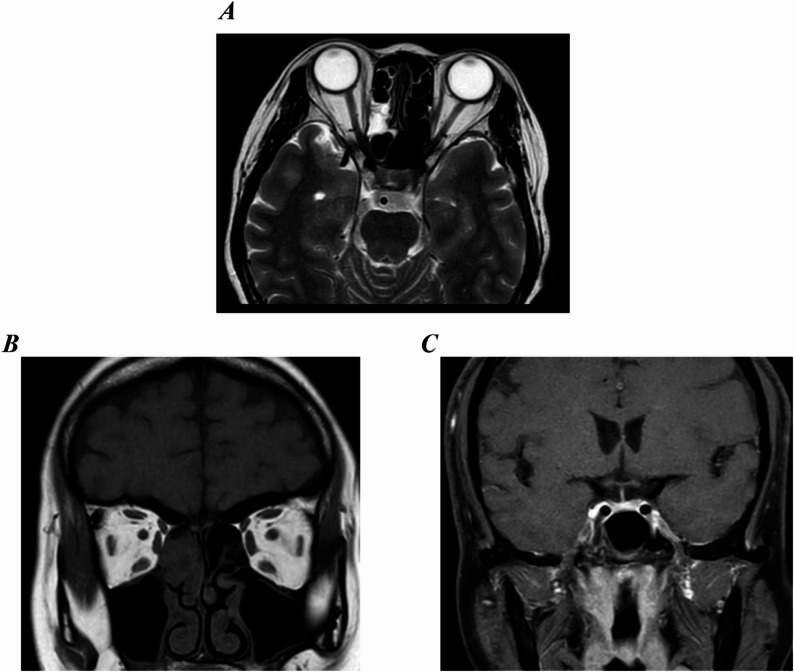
Neuroimaging of Patient 3. A: T2-weighted orbital MRI at initial presentation showing the left eye-globe in an adducted position. B: T1-weighted orbital MRI demonstrating normal extraocular muscles with no structural abnormalities. C: Gadolinium-enhanced T1-weighted MRI showing no abnormal enhancement of the cavernous sinus
